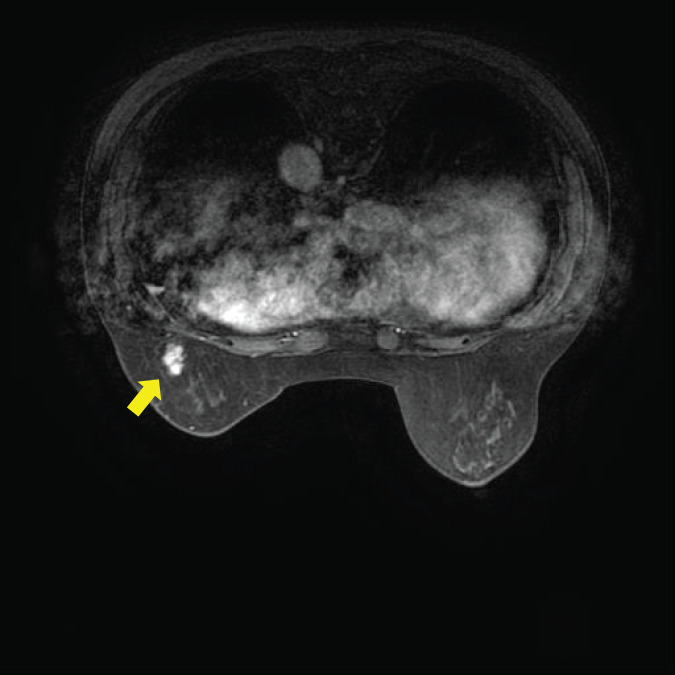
MRI findings. MRI revealed a 1.7-cm high-signal mass on DWI, which showed enhancement after contrast agent administration (yellow arrow). DWI, diffusion-weighted imaging
